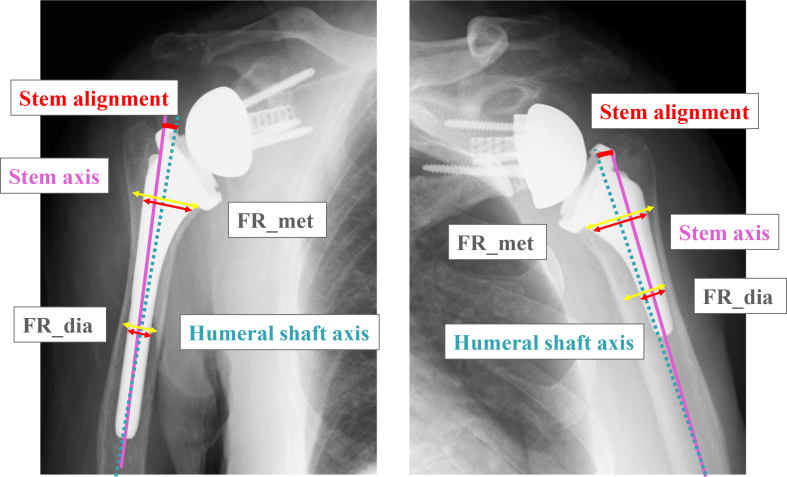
Radiographic parameters. The canal filling ratio was calculated as stem width divided by endosteal canal width at the metaphyseal (FR_met) and diaphyseal (FR_dia) levels. Coronal alignment was measured as the angle between the stem axis and humeral shaft axis.
